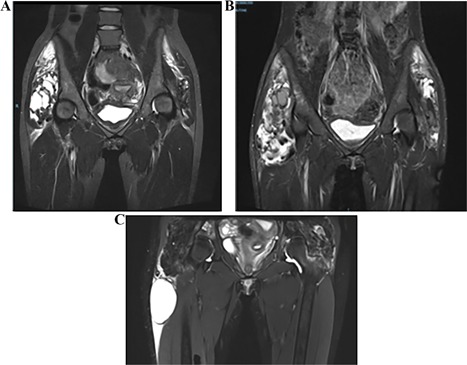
MRI comparison of the hips. (*A*) March 2016: right mass 106 × 121 × 65 mm, left mass 82 × 95 × 60 mm. (*B*) March 2017: right mass 170 × 121 × 65 mm, left mass 112 × 119 × 67 mm. (*C*) March 2018: calcic masses stable; occurrence of milky cyst in right thigh.
